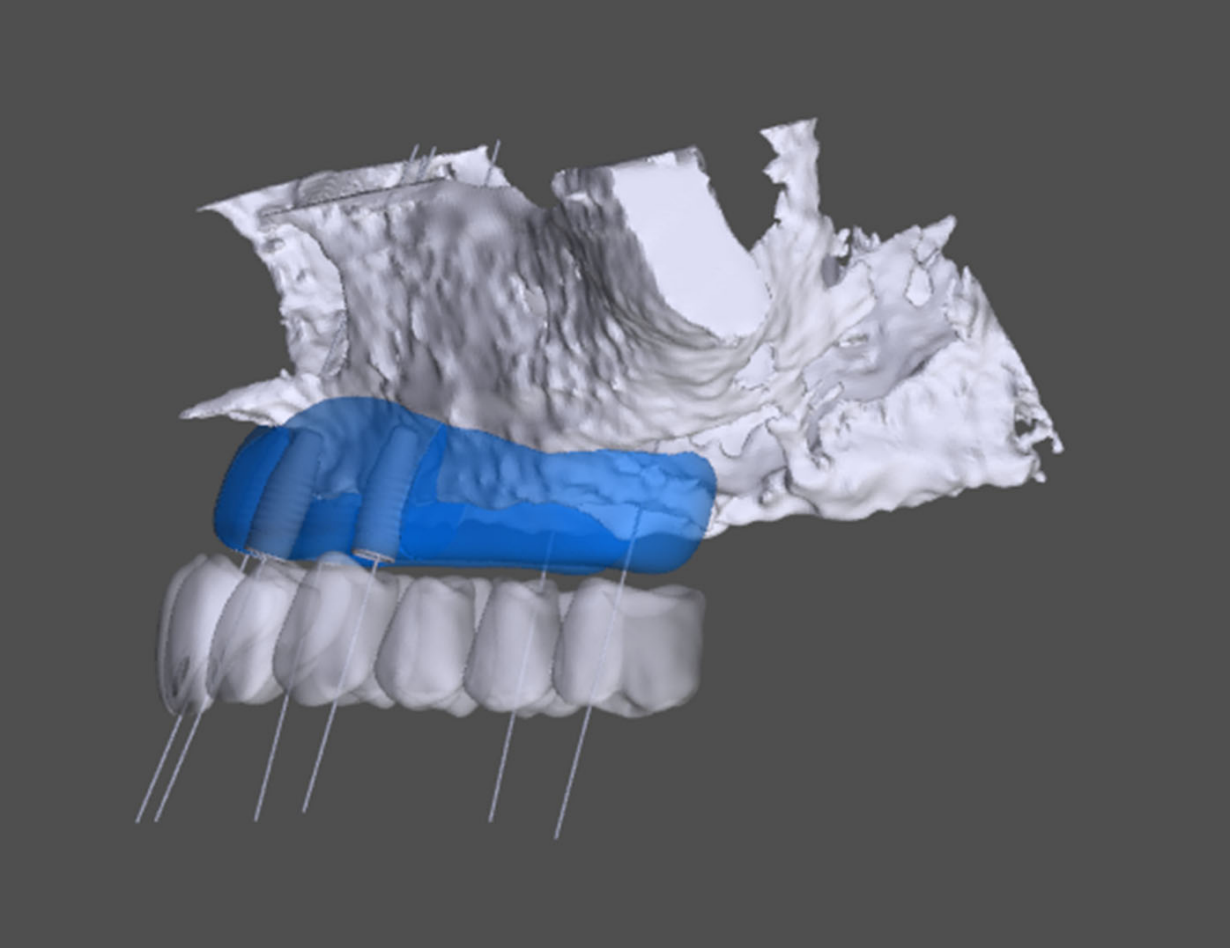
Virtual model of a CBCT for advanced backward planning for prosthetics and dental implants (grey) and customized milled Bonebuilder® for the maxilla (Blue; Botiss®-Software; Botiss-Straumann (CH-Basel). Lateral view
